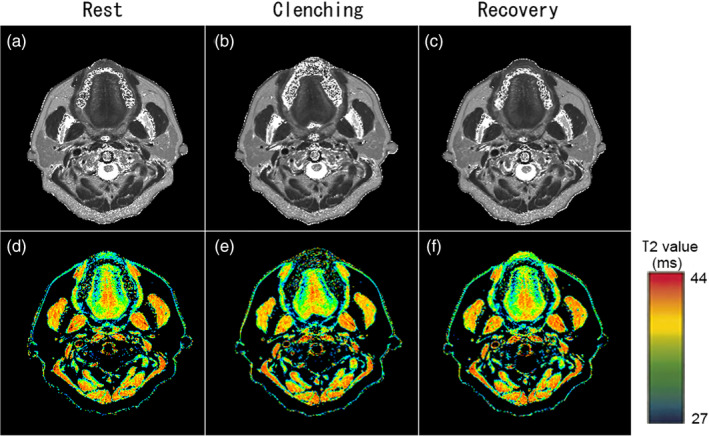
Representative axial T2‐weighted magnetic resonance images (a–c) and T2 mapping images (d–f)
